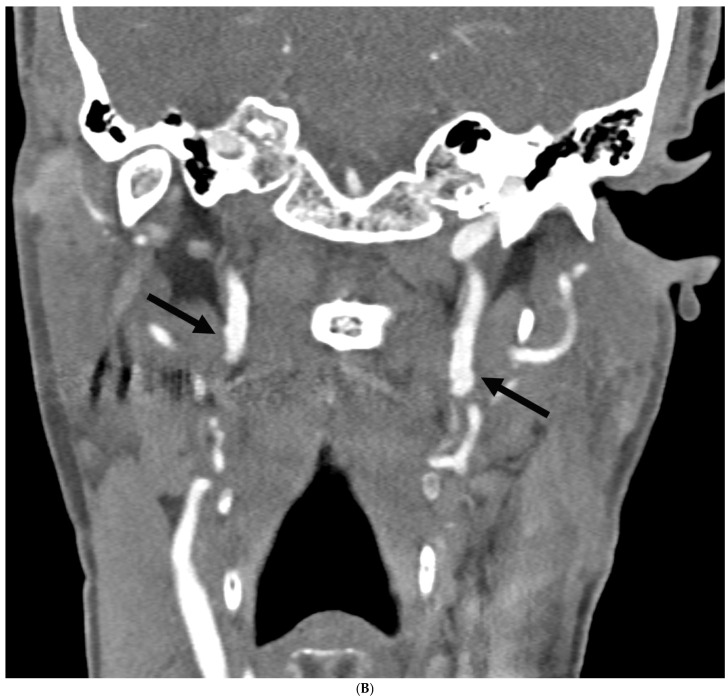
(A) 65-year-old woman with hEDS and a history of chronic migraine. Coronal CT angiography image demonstrates mild luminal irregularity (“beaded appearance”) of the V3 segments of the bilateral vertebral arteries (arrows), compatible with FMD. (B) 53-year-old woman with HSD and a history of episodic migraine. Coronal CT angiography image demonstrates a beaded appearance of the bilateral cervical internal carotid arteries (arrows), compatible with FMD.
